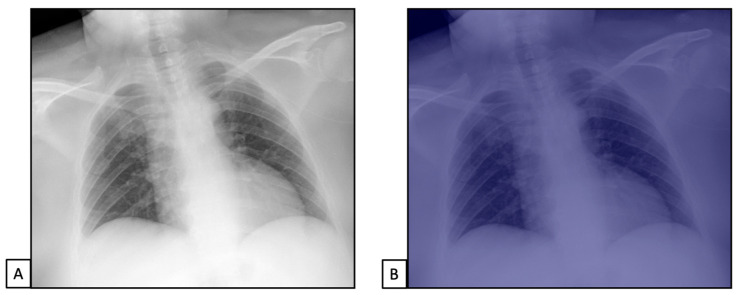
Chest X-rays of patients admitted to the emergency department with thoracic pain belonged to dataset 3. (A) represents CXR acquired at the bedside, showing no pathological findings. (B) represents AI system analysis, showing no pathological zones. The AI system reported a high suspicion of healthy subjects (98.5%). The final diagnosis was thoracic pain due to myocardial infarction.
